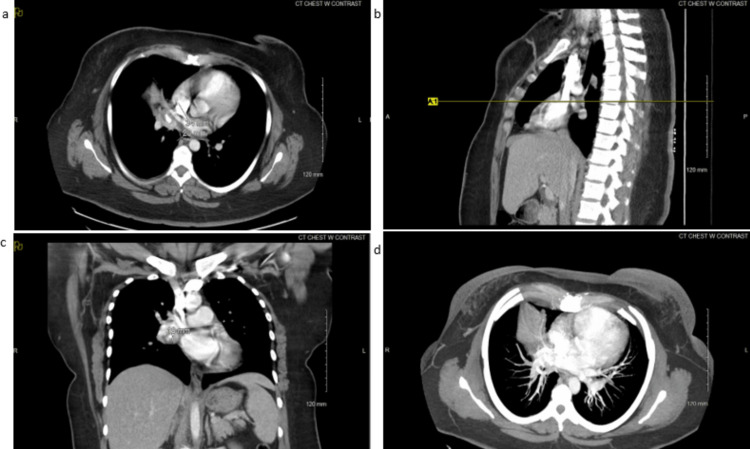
CT imaging of chest and lung from April 2023. (a) Mid-axial CT of the chest. The white radiological markings indicate the tumor area size. (b) Saggital CT of the chest. The yellow radiological marker indicates the tumor area of interest. (c) Coronal CT of the chest. The white radiological marker indicates the tumor area size. (d) Axial maximum intensity projection (MIP) CT of the lungs.
